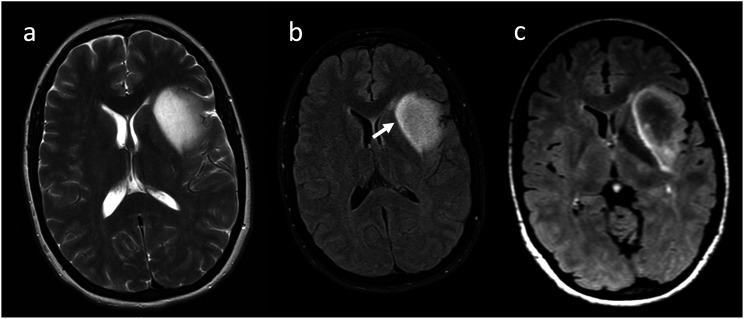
(a),(b) IDH-mutant, 1p/19q non-codeleted glioma with T2FMM in a 30-year-old female. T2WI (a) shows a left insular mass with homogenous T2 signal, and the FLAIR sequence (b) shows subtle hypointense signal relative to a thin peripheral hyperintense rim. The hyperintense rim is only appreciated on the medial aspect of the lesion (arrow). (c) 3 weeks later a repeat MRI was performed using a different scanner. The relative hypointensity is much more evident on this study, and the thin hyperintense rim is circumferential. The acquisition information for the images “b” versus “c” were as follows: magnetic field strength of 1.5 versus 3 T, Echo Time of 124 versus 91 ms, Inversion Time of 2500 versus 2098 ms. Image “b” was obtained via 2D acquisition with fat saturation, and image “c” via 3D acquisition without fat saturation.
